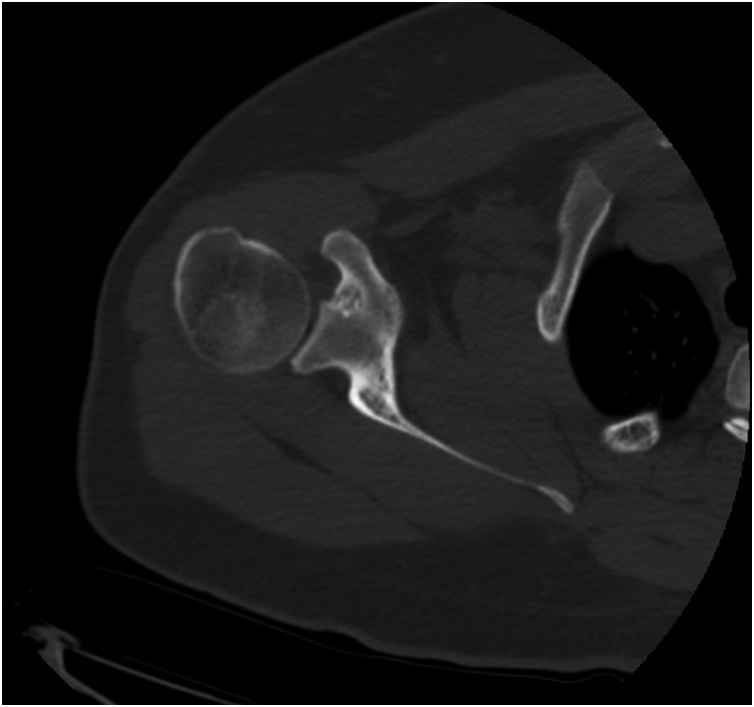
Contrast-ehanced axial CT of the right shoulder.
